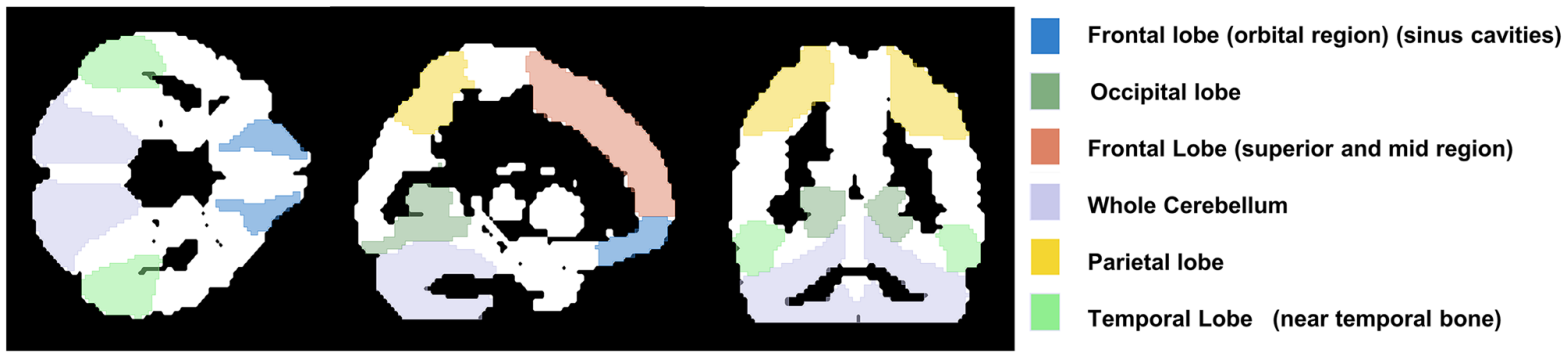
VOIs for regional quantification of FDG PET images
